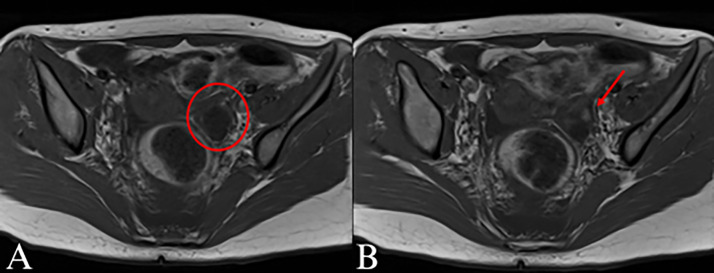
(A) Axial T1-weighted magnetic resonance imaging (MRI) of the pelvis shows the left ovary (red circle) (B) containing a hyperintense focus relative to surrounding tissue measuring up to 9mm (red arrow). In addition to an ovarian teratoma, the differential diagnosis of this could include a proteinaceous cyst, hemorrhage or fat.
